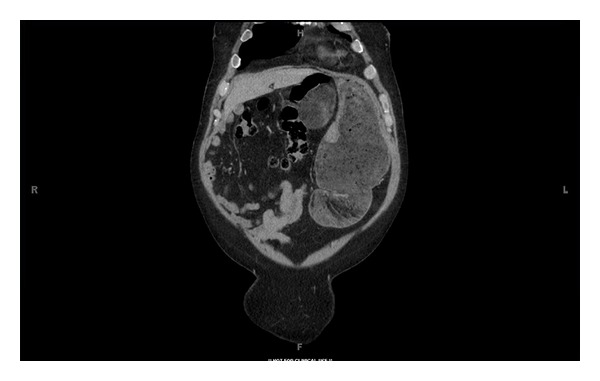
Computed tomographic coronal image showing epigastric mass abutting dilated stomach and duodenum.
